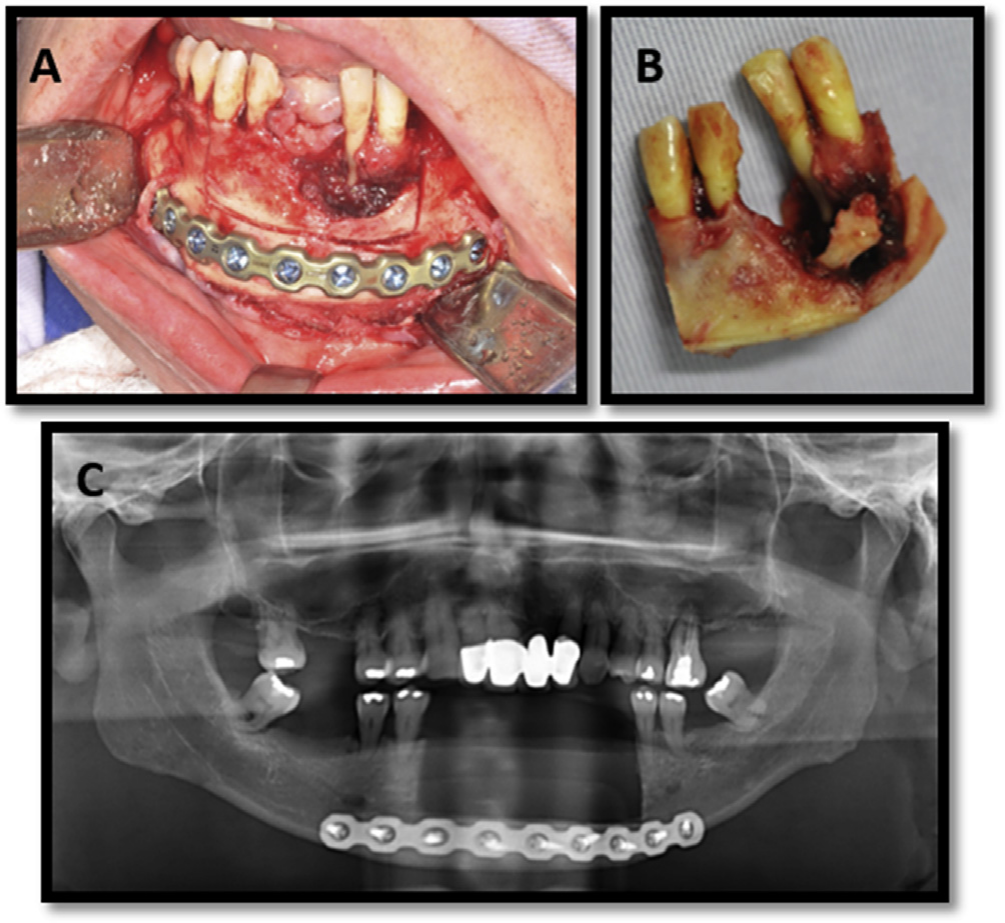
A. – Demarcation of the resected area and stabilization of the remaining bone using a 2.4-mm system fixation plate. B. Removed section. C. Post-surgery panoramic radiography confirming stability of the internal fixation.
